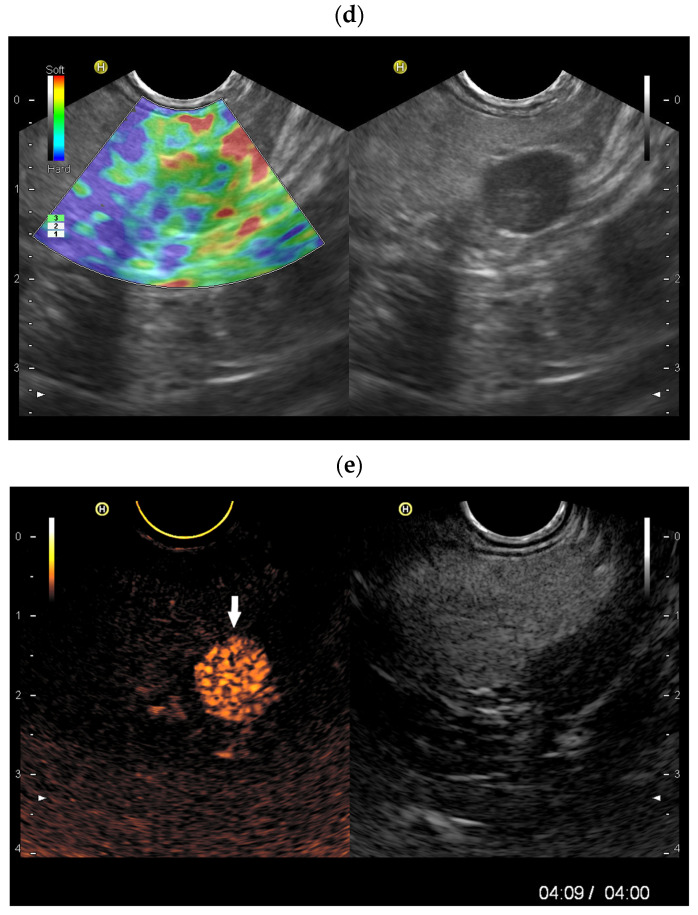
Intrapancreatic accessory spleen. CT scan performed due to abdominal complaints. The CT scan showed a hyperenhanced lesion in the tail of the pancreas. Correspondingly, EUS revealed a smooth-bordered, approximately 11 mm, slightly hypoechoic lesion in the tail of the pancreas (arrow) (a). Feeding vessel (arrow) (b) and central vessels (c) were visible. Elastography showed that the lesion was not stiffer than the surrounding pancreatic tissue (d). CE-LMI-EUS (SonoVue) demonstrated a hyperenhanced lesion. The examination was terminated after four minutes; the lesion (arrow) remained intensively hyperenhanced (e). These findings are typical of an intrapancreatic accessory spleen. EUS-FNA was thus not performed. Further investigations and follow-up were considered unnecessary owing to the highly specific findings.
